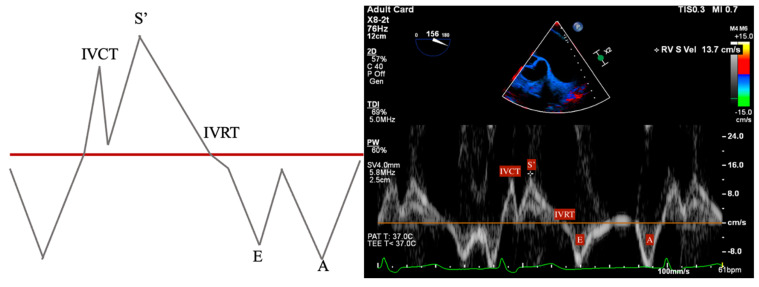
Tissue Doppler Imaging (TDI) of lateral tricuspid valve annulus in DTG RV Inflow/Outflow view. Isovolumetric contraction time (IVCT) and isovolumetric relaxation time (IVRT).
